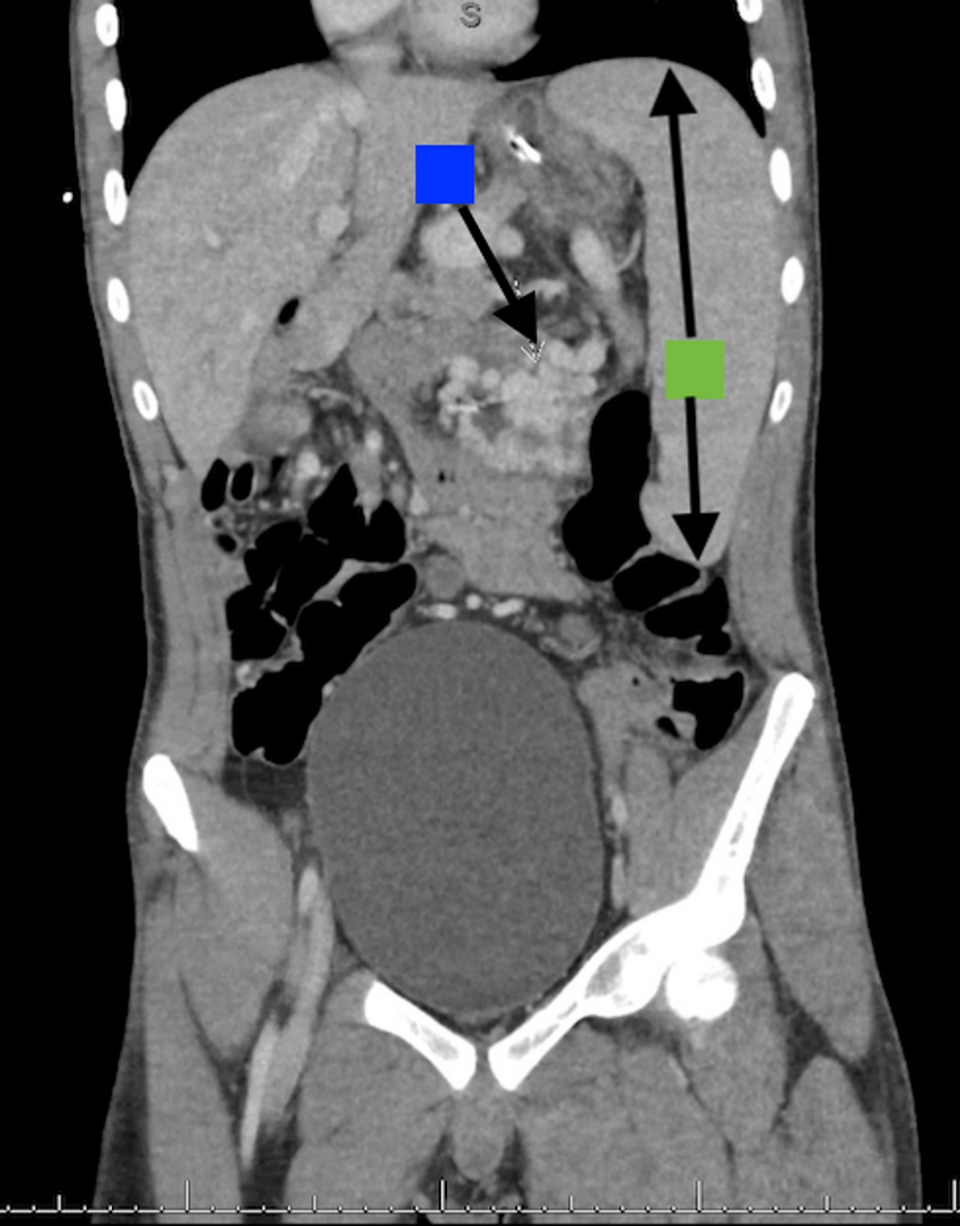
CT angiogram of the abdomen showing massive jejunal varices (blue arrow), and massive splenomegaly (green bidirectional arrow) of a craniocaudal length of 20 cm CT: computed tomography
